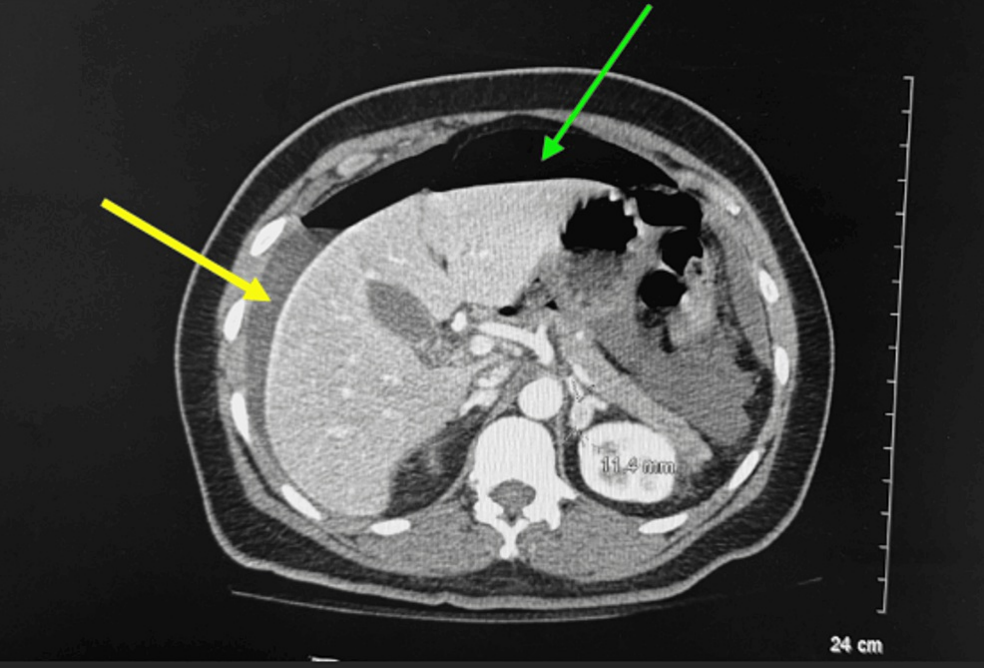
CT scan of the pelvis and abdomen with IV contrast showing free intraperitoneal air (represented by the green arrow) and free fluid (represented by the yellow arrow) suspicious of bowel perforation with focal wall thickening along the gastric pylorus. Findings suspicious for CT hypoperfusion.
